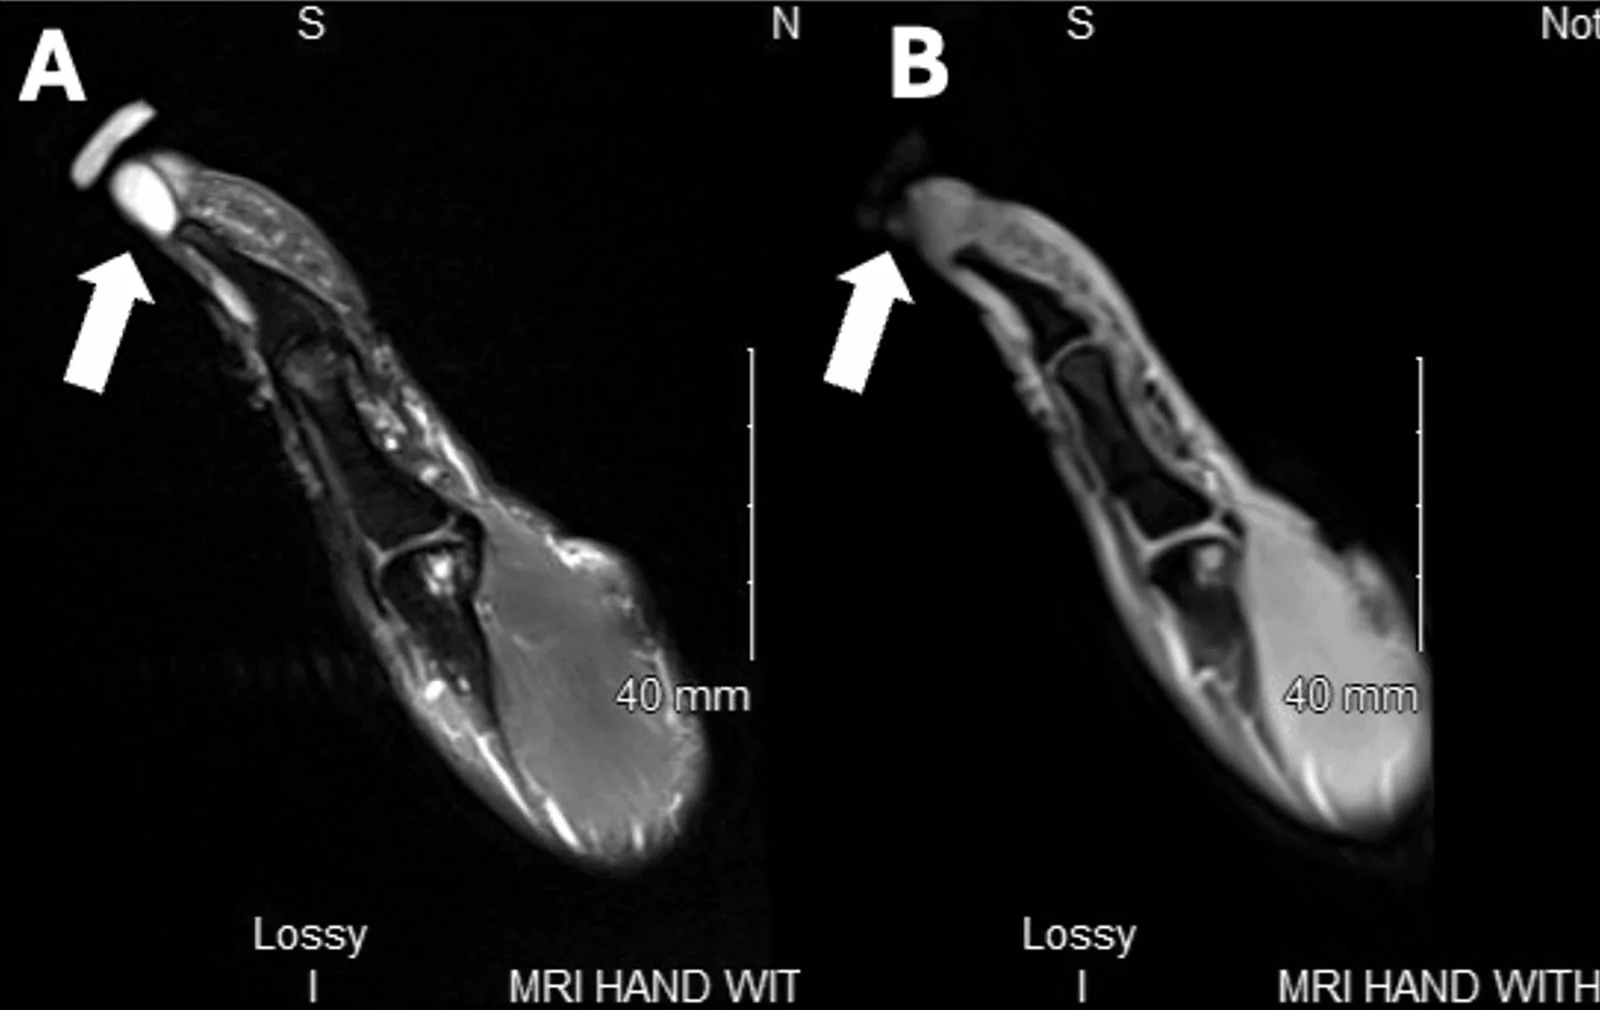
Sagittal precontrast (A) and postcontrast (B) T1-weighted VIBE magnetic resonance images of the right thumb demonstrating the lesion within the thumb pulp without significant postcontrast enhancement. VIBE: volumetric interpolated breath-hold examination
